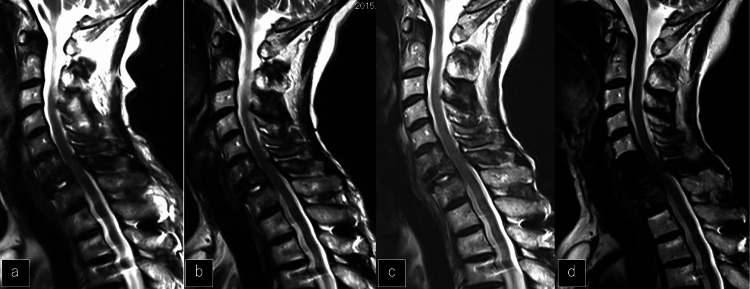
Changing image over time on MRI for five years a, b, c, d: Sagittal MRI at 3 months, 1 year, 2 years, and 5 years. Spinal cord decompression was maintained for 5 years.
